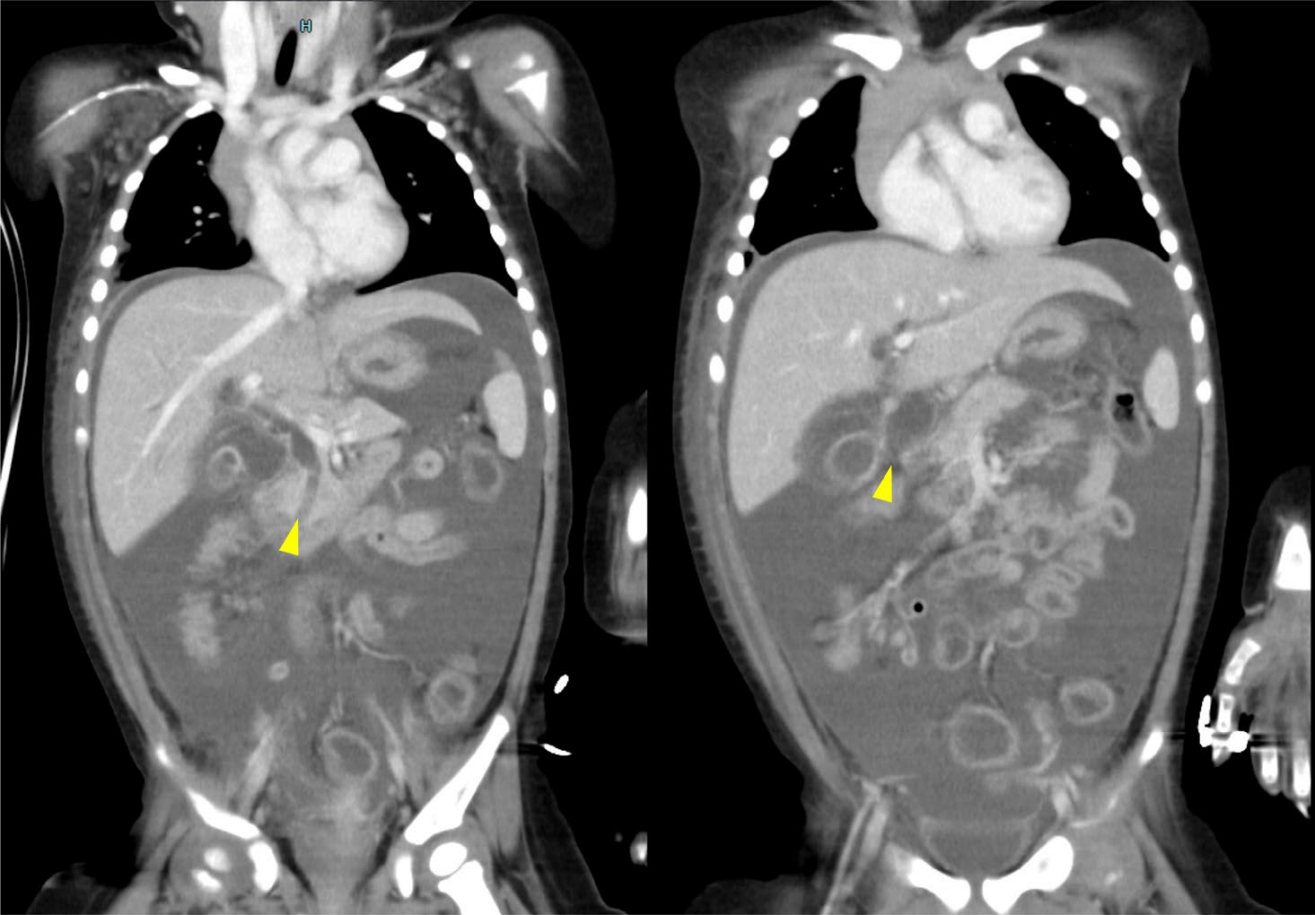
Preoperative contrast‐enhanced computed tomography. Contrast‐enhanced computed tomography (CT) revealed massive ascites, marked dilation of both intrahepatic and extrahepatic bile ducts, and a 19 mm‐long common channel containing a hyperdense structure suggestive of a protein plug (the left arrowhead). A discontinuity in the anterior wall of the common bile duct is indicative of perforation (the right arrowhead).
